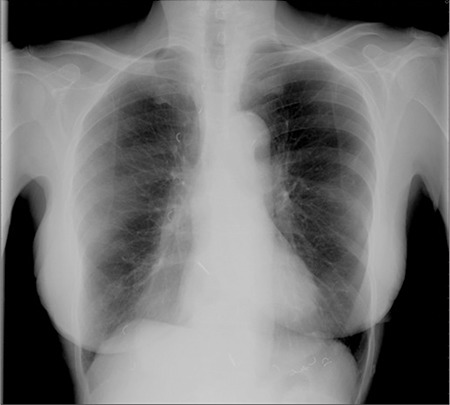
Frontal chest radiograph showing oesophageal conduit withmultiple surgical clips in the mediastinum to the right of midline.
